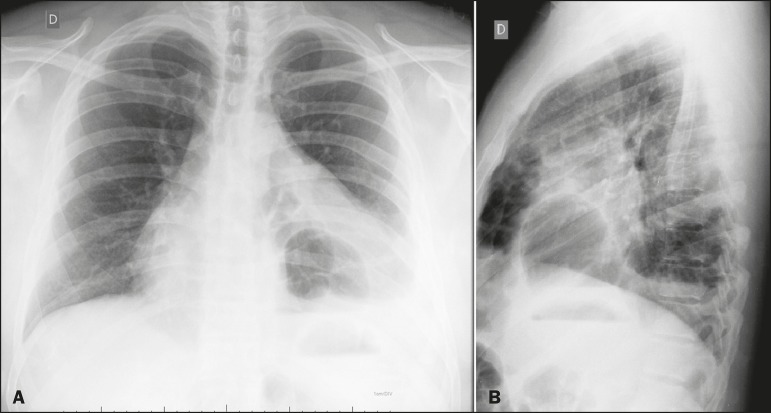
Posteroanterior and lateral X-rays of the chest (A and B, respectively)
